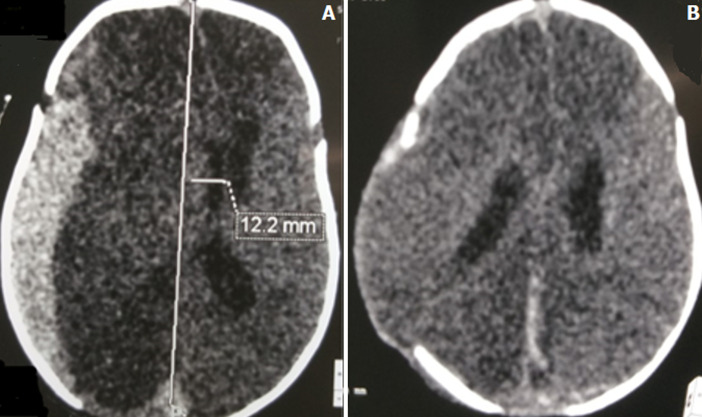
A) axial section brain CT scan of an HSDA and post ventricular puncture ischemia; B) cerebral CT scan axial section after decompressive HSDA and post-ventricular puncture ischemia
